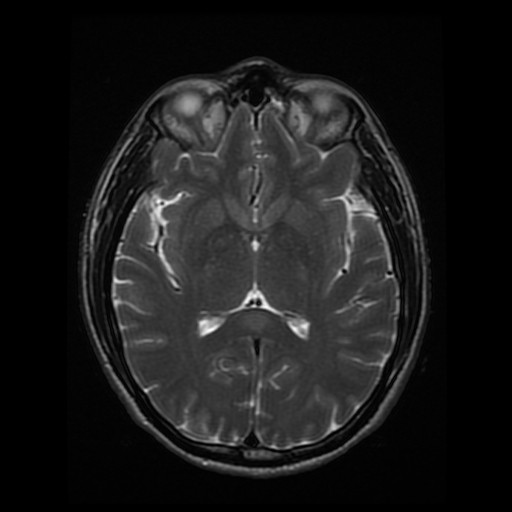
Axial T2-weighted MRI without contrast demonstrating focal hyperintensity of the SCC.
